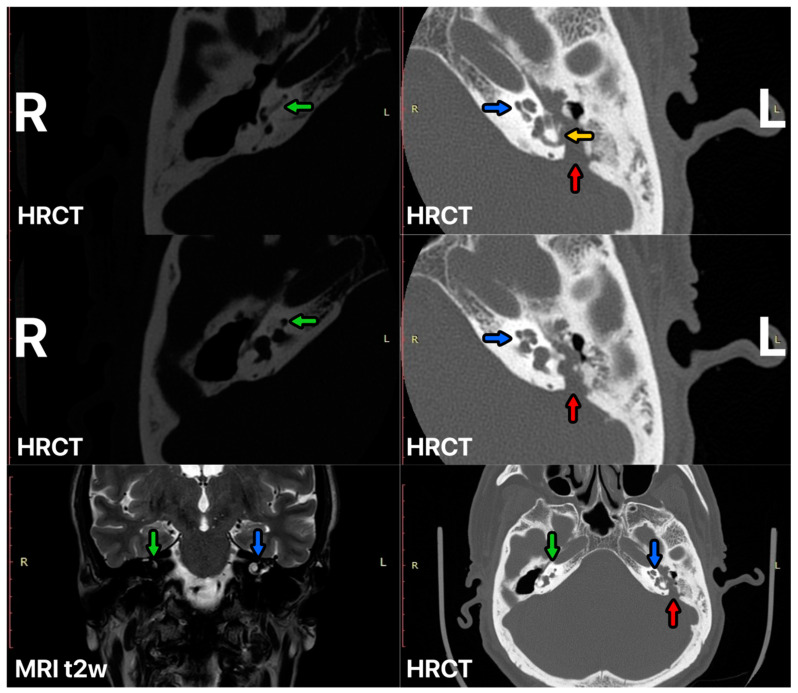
HRCT and MRI of the ear before SP surgery. Right cochlear ossification—green arrow, left cochlear—blue arrow, posterior fossa destruction by cholesteatoma—red arrow, lateral semicircular canal destruction—yellow arrow.
